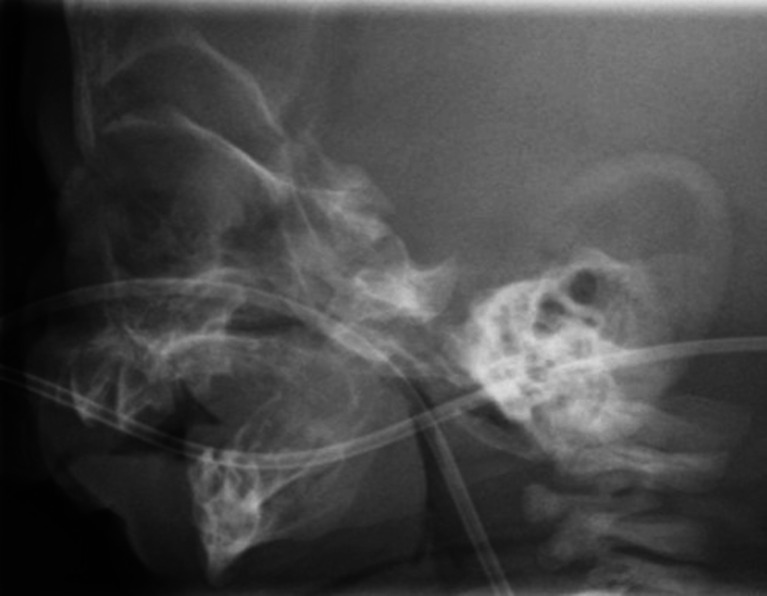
Example of micrognathia seen on lateral X-ray
